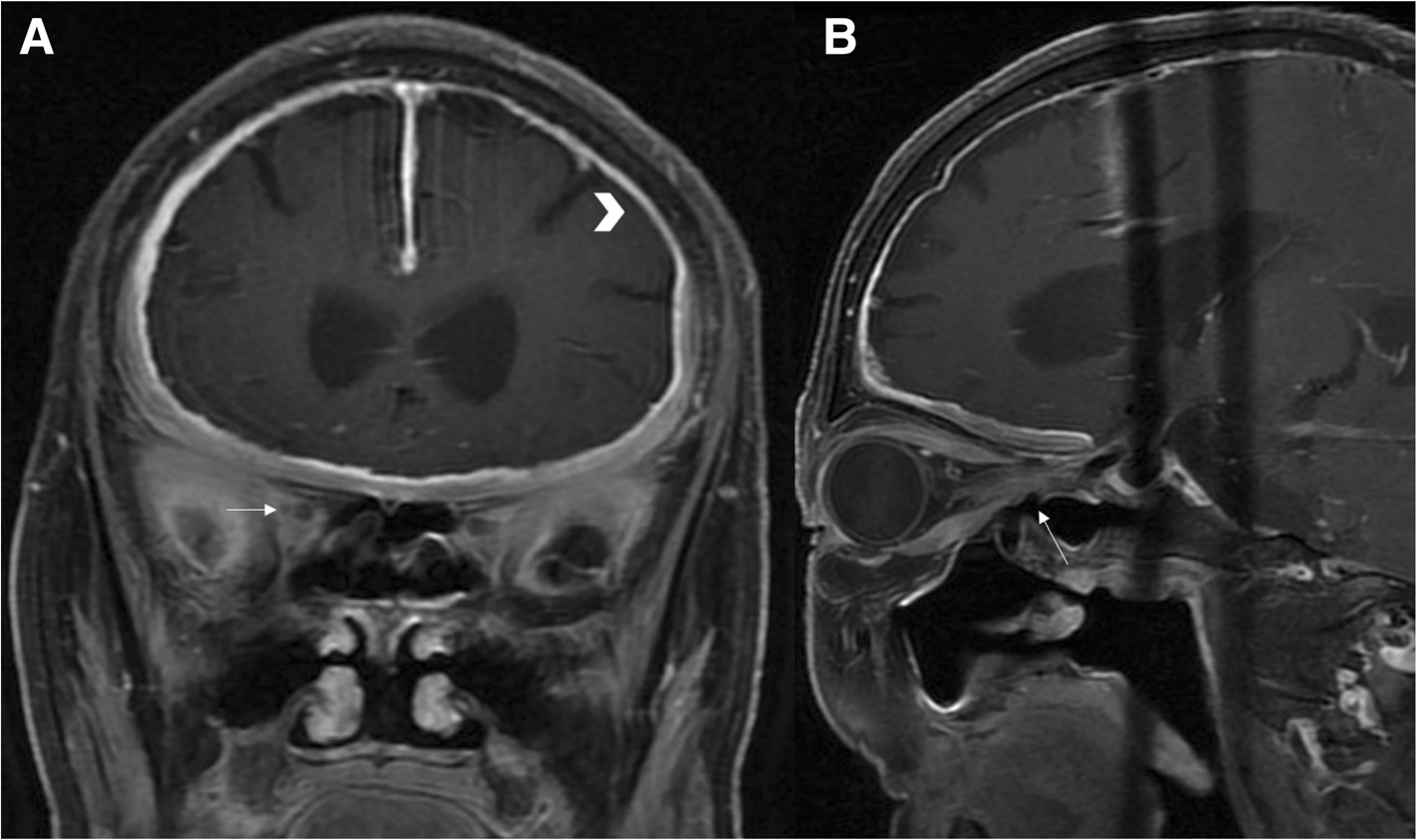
Compression of optic nerve as hypertrophic cranial pachymeningitis. Coronal contrast enhanced T1-weighted MRI (a) showing hyperintense linear dura thickening (arrow heads). Sagittal contrast enhanced T1-weighted MRI (b) showing the thickened dura mater was markedly enhanced after contrast media, and compressed the optic nerve in the orbit apex (white arrow)
